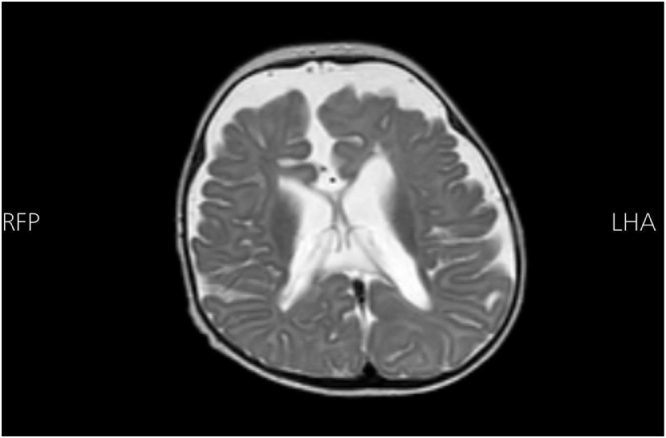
Brain MRI without contrast demonstrates partial agenesis of the corpus callosum, characterized by absence of the posterior body and splenium, while the anterior portion appears preserved. There is mild dilation of the lateral ventricles, predominantly involving the occipital horns (colpocephaly), consistent with secondary changes related to the callosal anomaly. The remaining cerebral structures, including the cortex, basal ganglia, thalami, and posterior fossa, show normal signal intensity and morphology, with no evidence of mass effect, hemorrhage, or abnormal enhancement.
